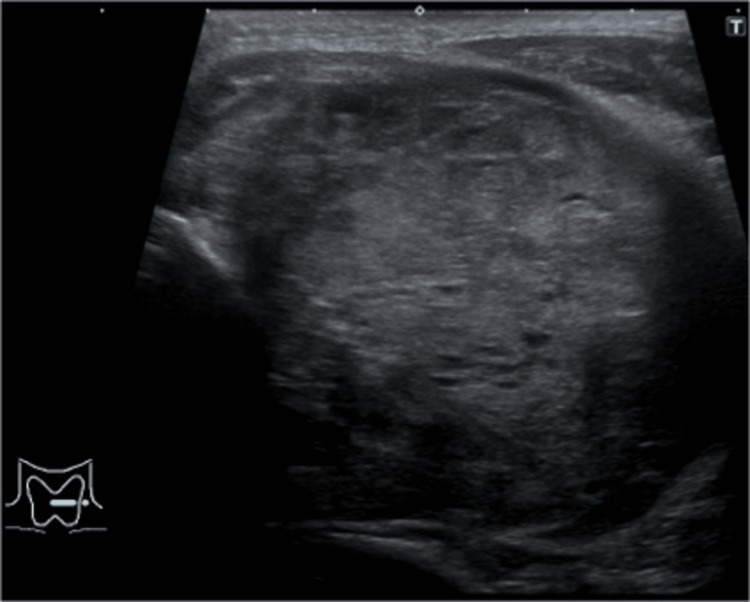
Cervical ultrasonography of the left thyroid lobe. In the left lobe of the thyroid, a solid nodule was observed with irregular shape, indistinct margins, and slightly heterogeneous internal hypoechogenicity.
